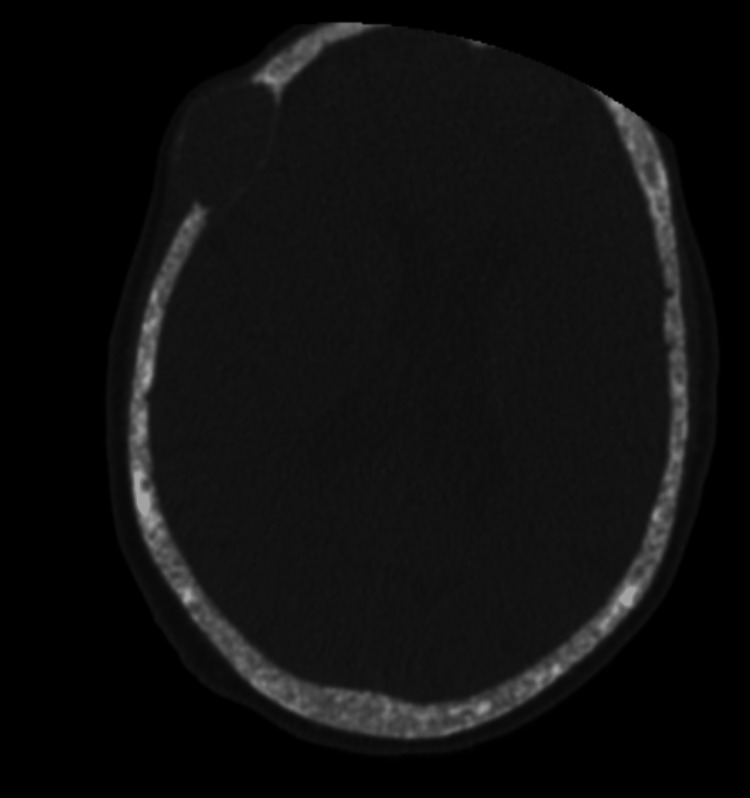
Salt and pepper skull on CT.
